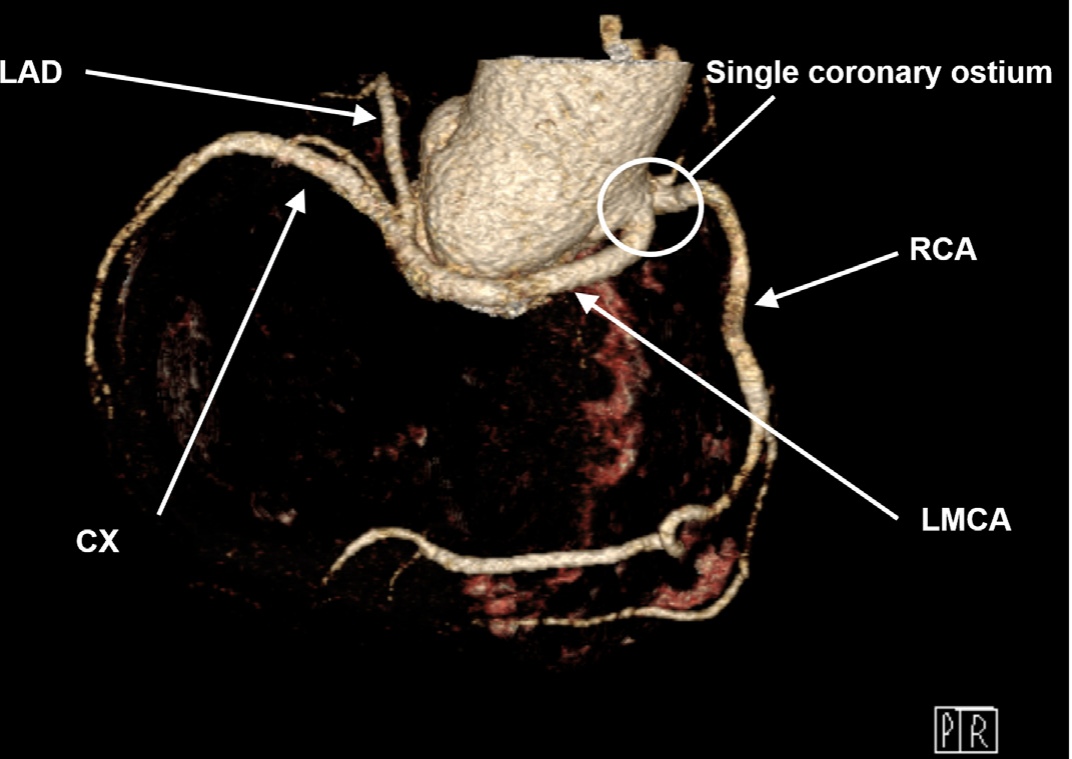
3-Dimensional Reformation of the Coronary Arteries in the Preoperative Computed Tomography Scan Single coronary ostium in the right sinus and long course of the LMCA along the right circumference of the aorta. CX = circumflex artery; LAD = left anterior descending artery; LMCA = left main coronary artery; RCA = right coronary artery.
